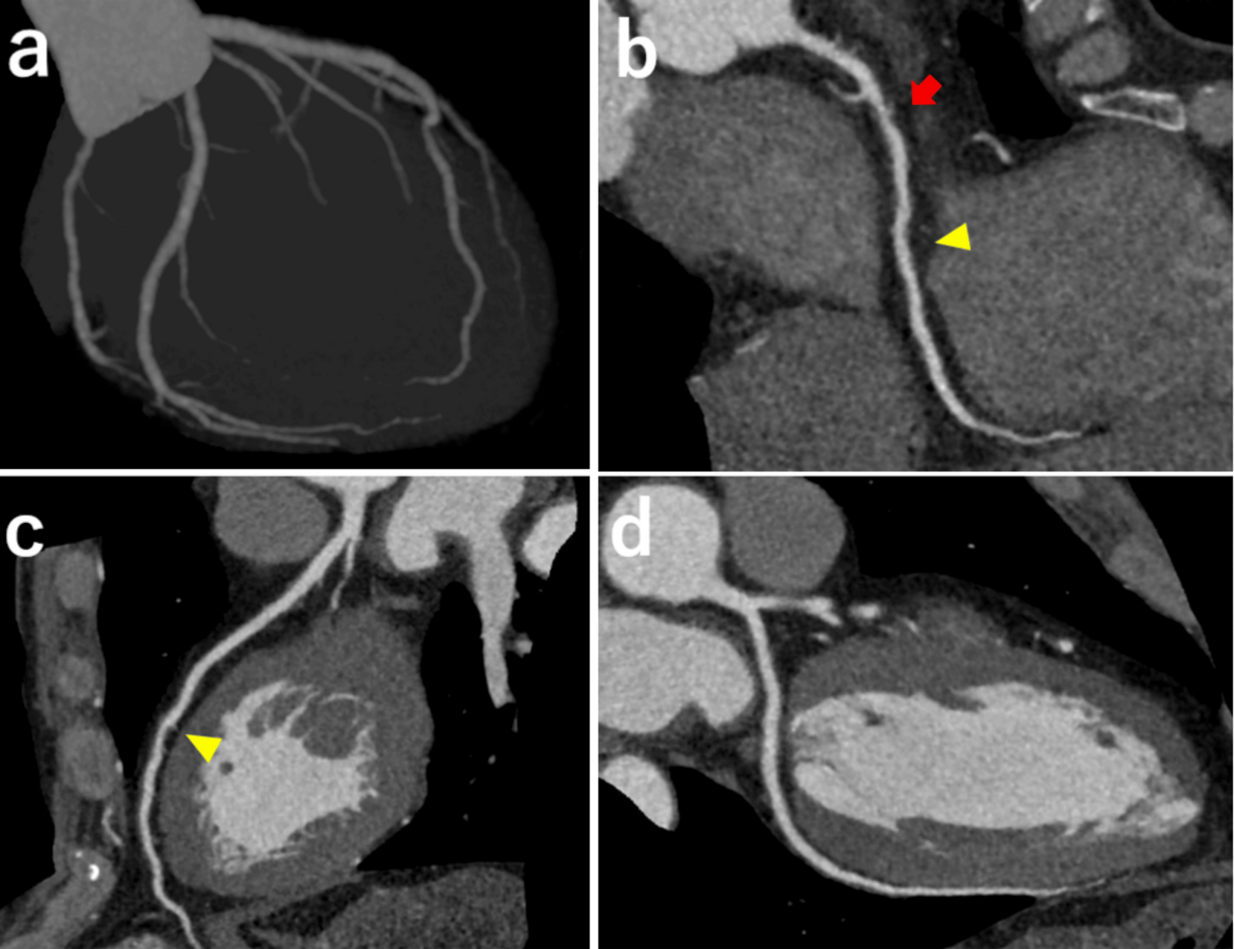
Coronary CT angiography (a) Overall view, (b) Right coronary artery — moderate stenosis in segment #1 (arrow) and mild stenosis in #2 (arrowhead), (c) Left anterior descending artery — mild stenosis in #7 (arrowhead), and (d) Left circumflex artery — no remarkable stenosis
